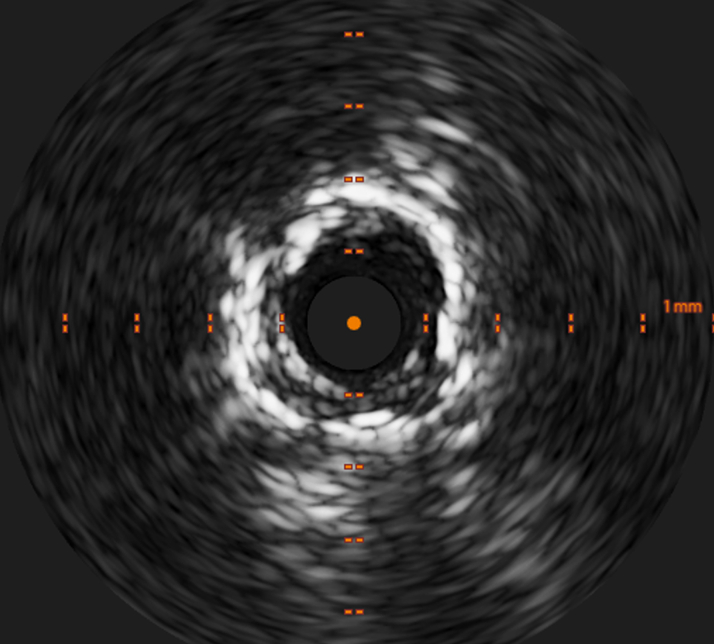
Intravascular Ultrasound Image Showing Underexpansion and Neointimal Hyperplasia Within the Stented Segment
